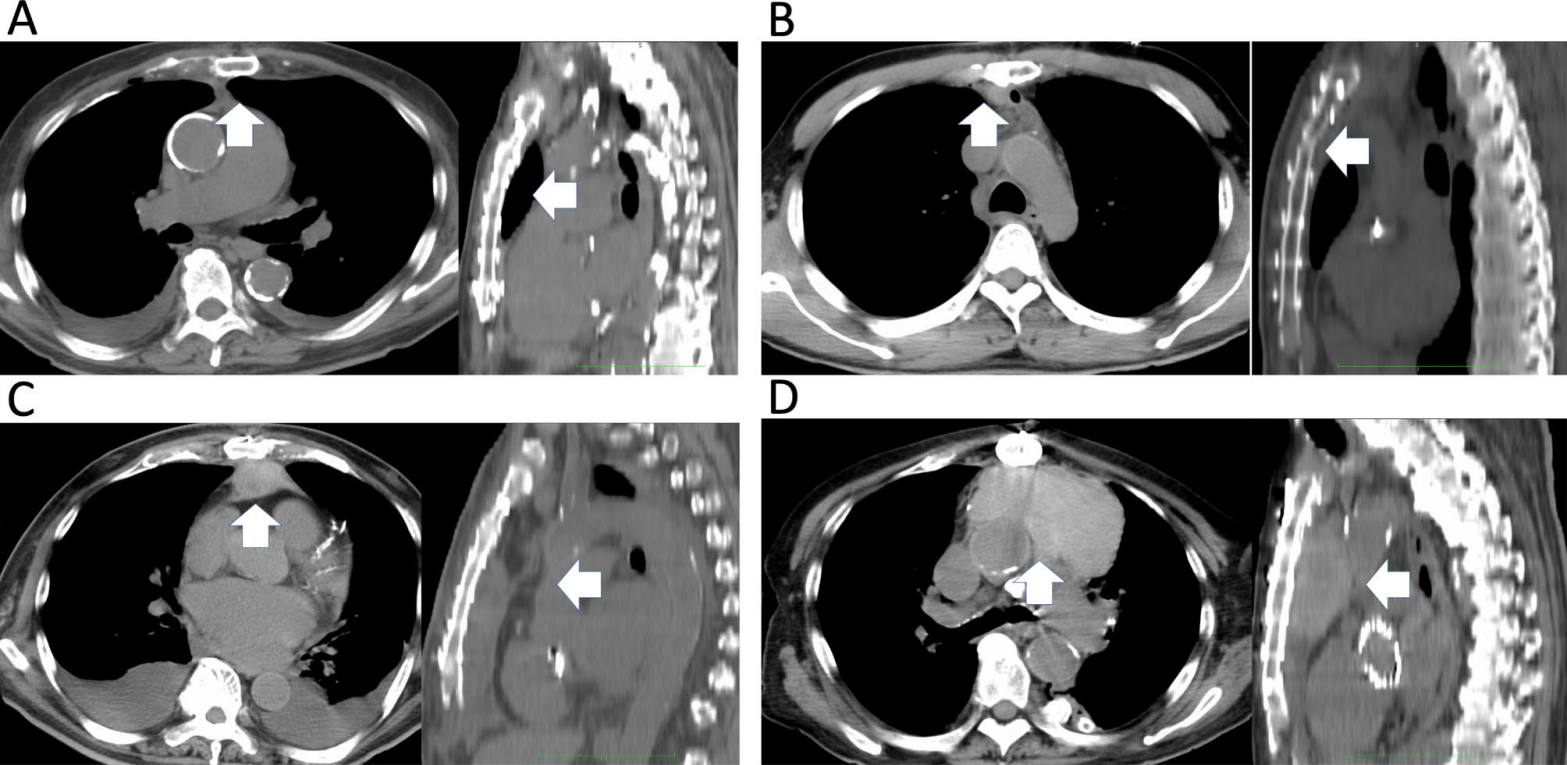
Grade of substernal hematoma. A) Substernal hematoma is not present on CT. B) Slight substernal hematoma is seen on CT. C) Substernal hematoma equal to or larger than the size of the sternum, up to twice the size. D) Substernal hematoma larger than twice the size of the sternum. CT, computed tomography
